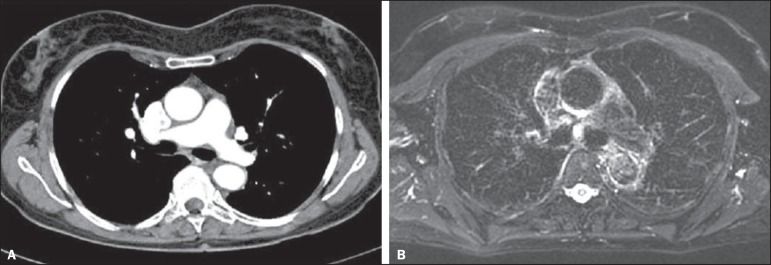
A: Axial CT image showing a 8 mm lymph node in the subcarinal
